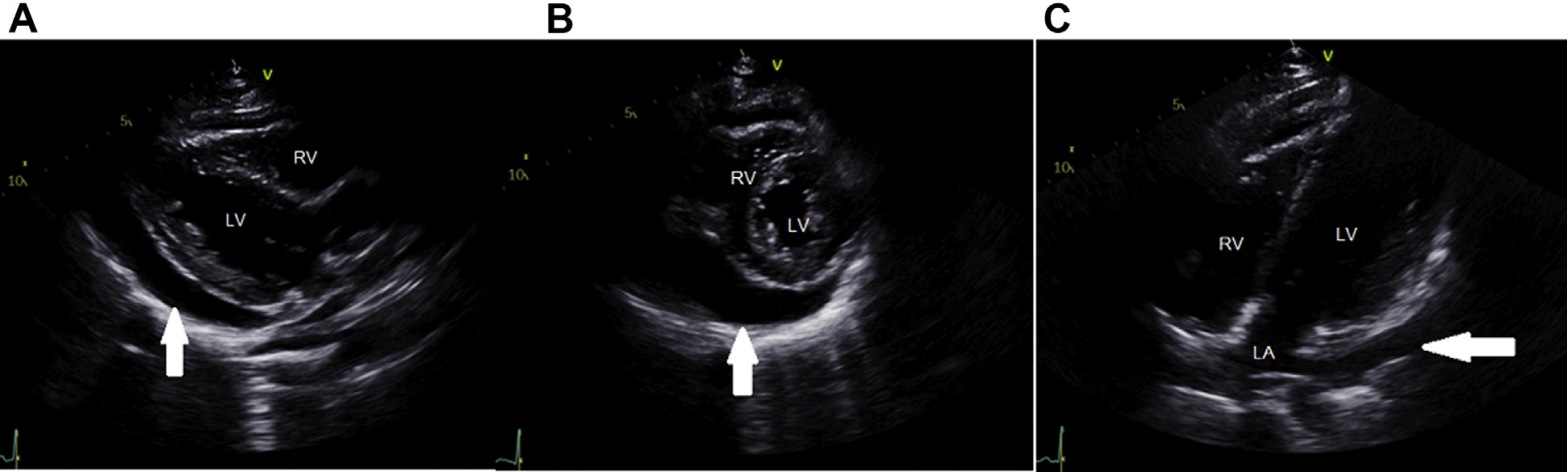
Repeated Echocardiography Repeat (A) parasternal long-axis, (B) parasternal short-axis, and (C) off-axis apical views. The pericardial effusion has increased in size (arrows). Abbreviations as in Figure 1.
